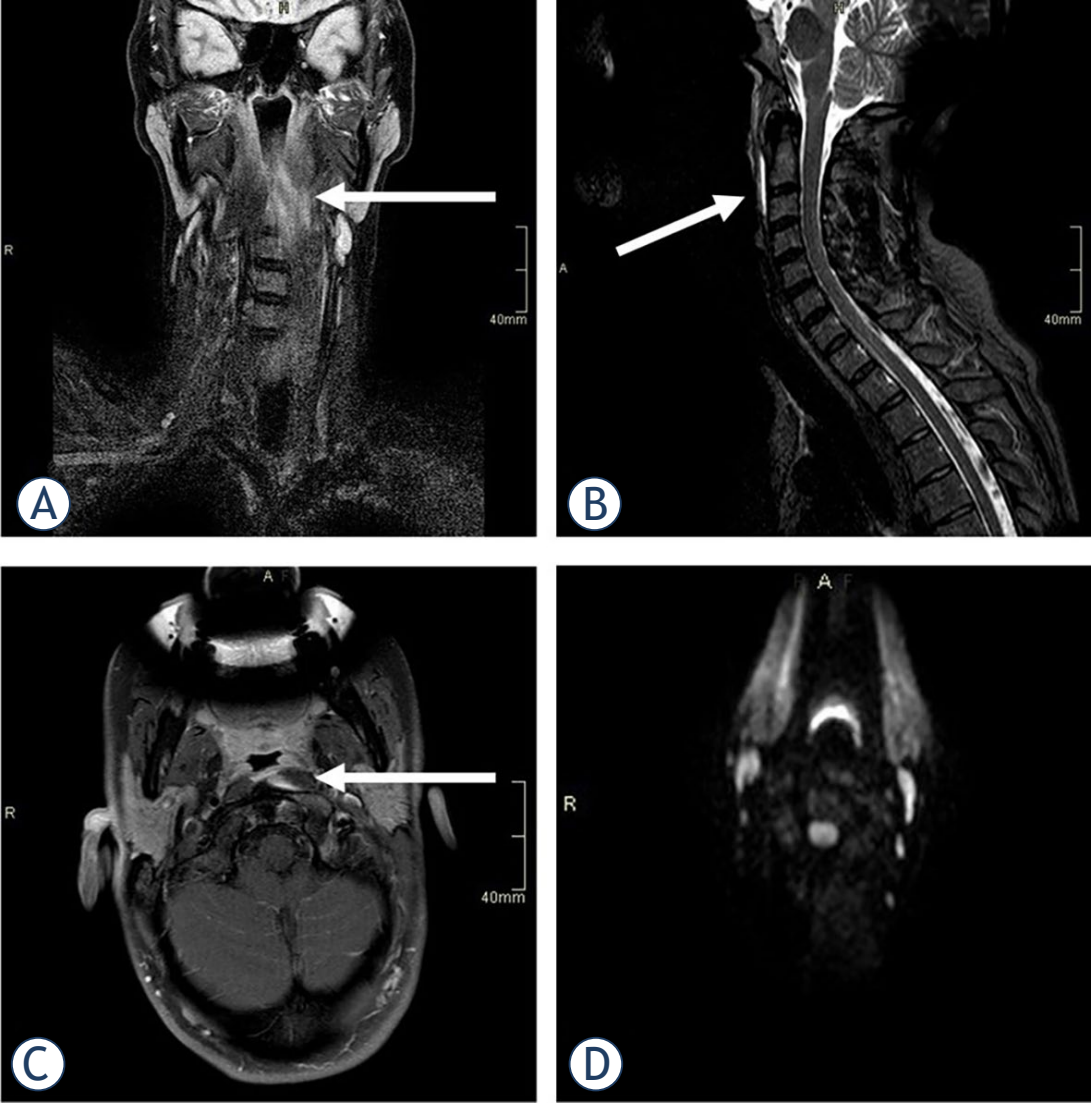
MRI in a 40-year-old female showed short tau inversion recovery sequence (STIR) hyperintensity in the upper part of her left Longus colli muscle, suggesting an oedema (A), with thin prevertebral effusion on sagittal images (B). After intravenous gadolinium contrast injection, a small area of enhancement was observed in the medial aspect of the muscle (C), but no peripherally enhancing collection to suggest an abscess was present. Diffusion-weighted imaging was normal, excluding the presence of pus (D).
